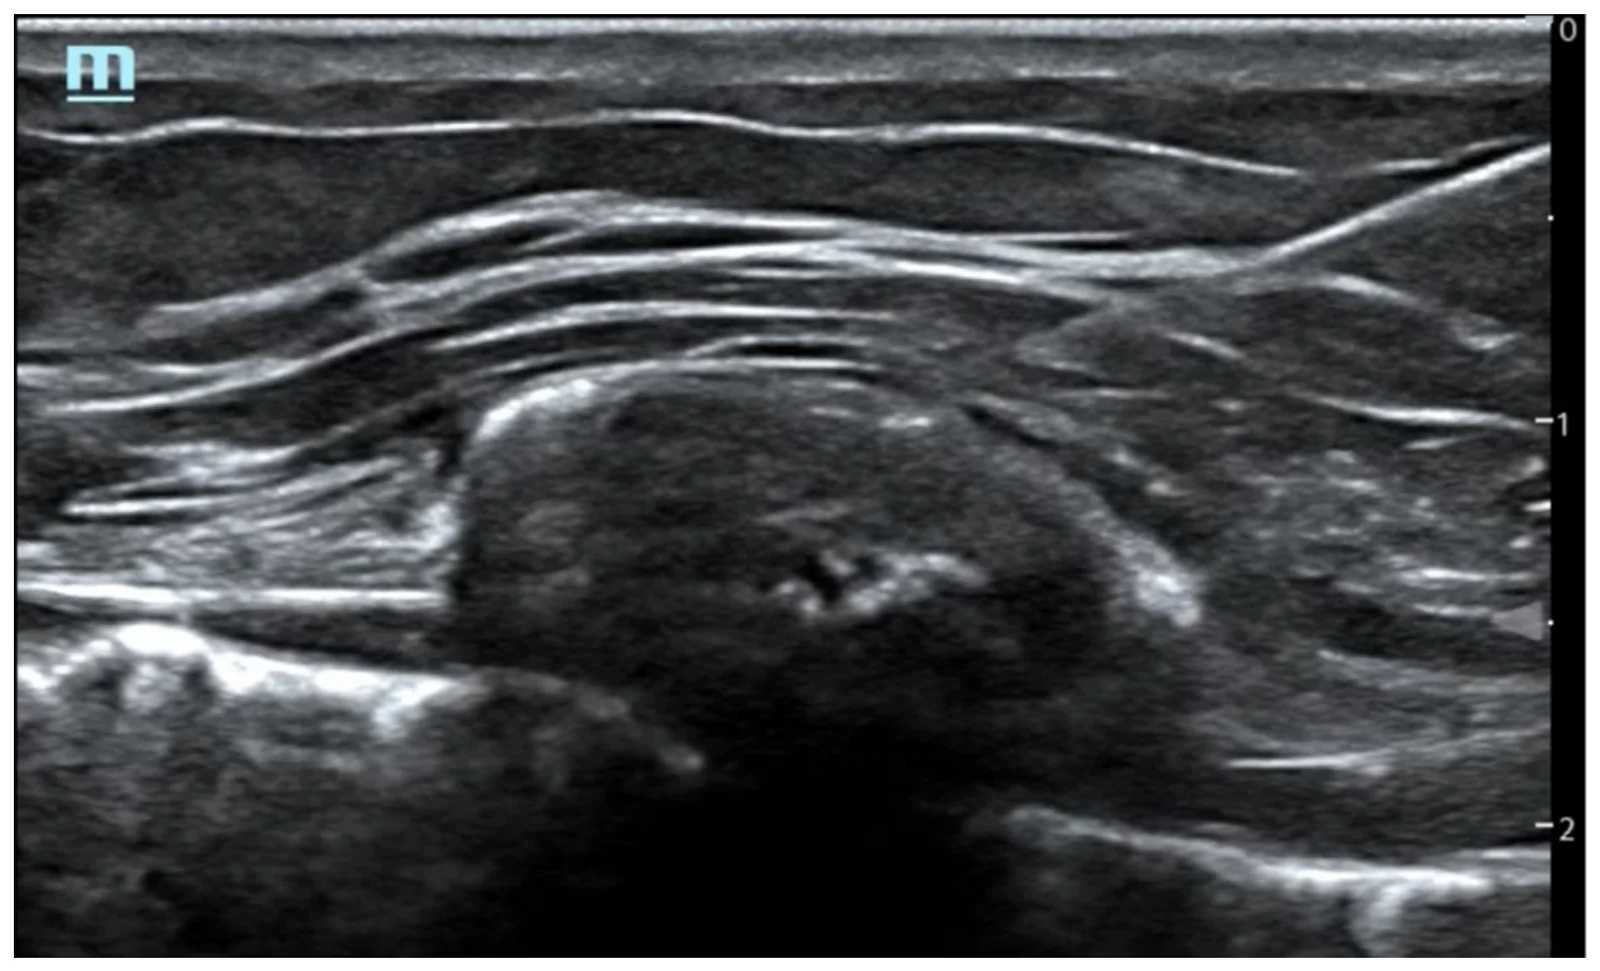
Ultrasound view of hydrodissection during modified SPIP block.
